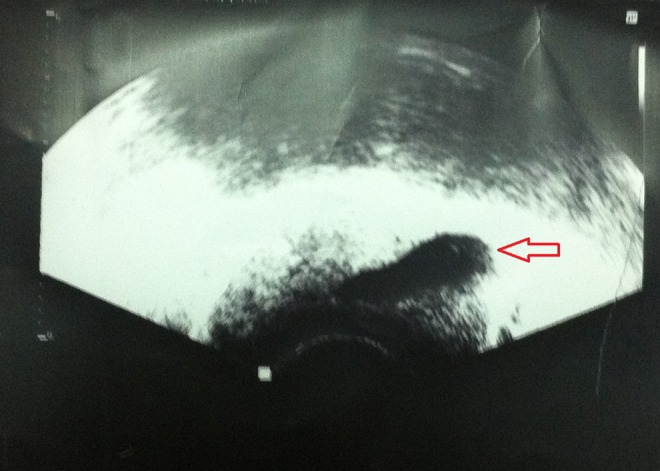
TRUS showing a tubular cystic structure inserting to the site of left ED associated with prominent terminal part of vas deferens (left EDO). ED, ejaculatory duct; EDO, ejaculatory duct obstruction; TRUS, transrectal ultrasonography.
